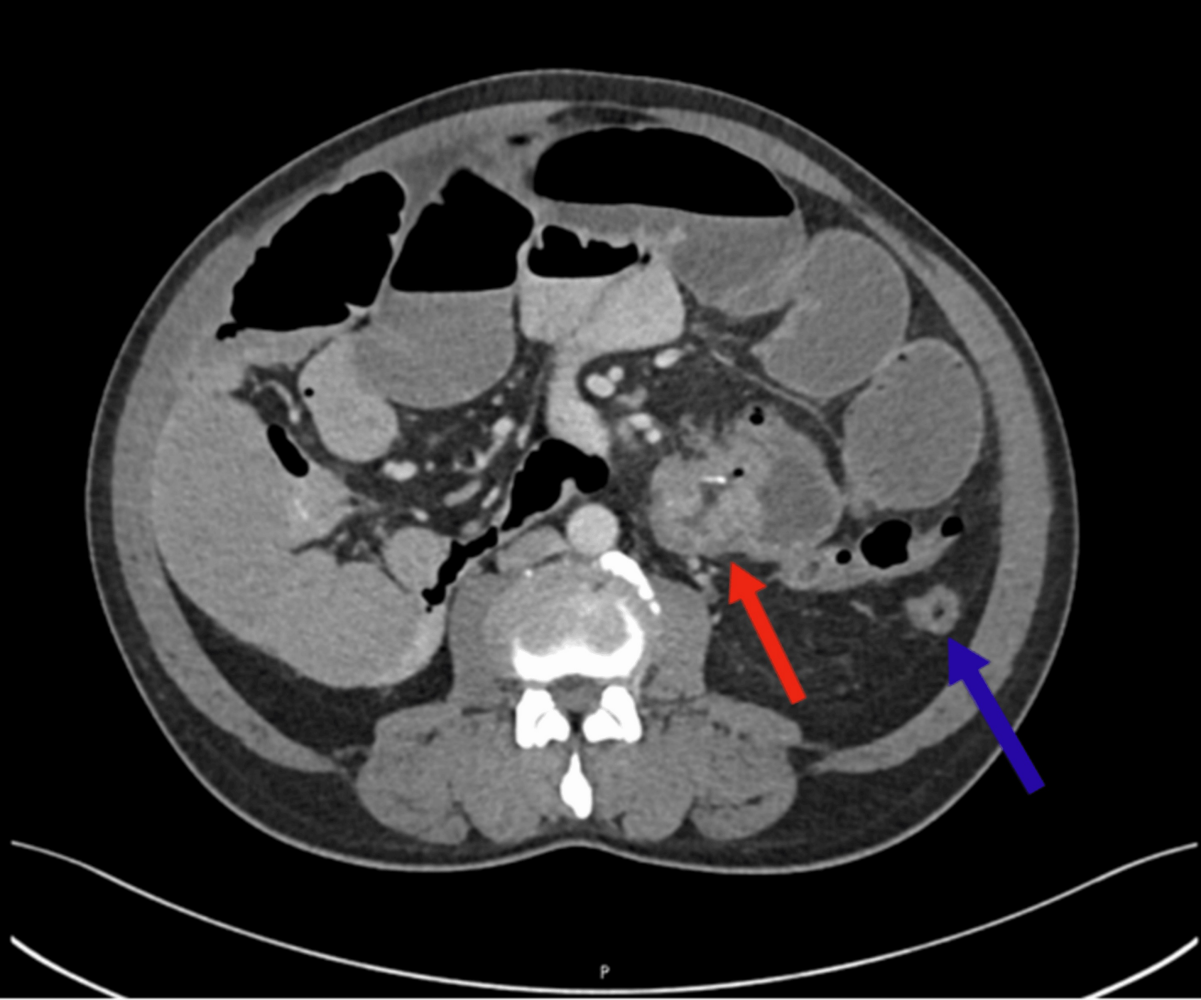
Axial view on CT of the abdomen showing both the obstructing ascending colon tumor (red arrow) and descending colon (blue arrow) on the left side of the abdomen. CT: computed tomography
